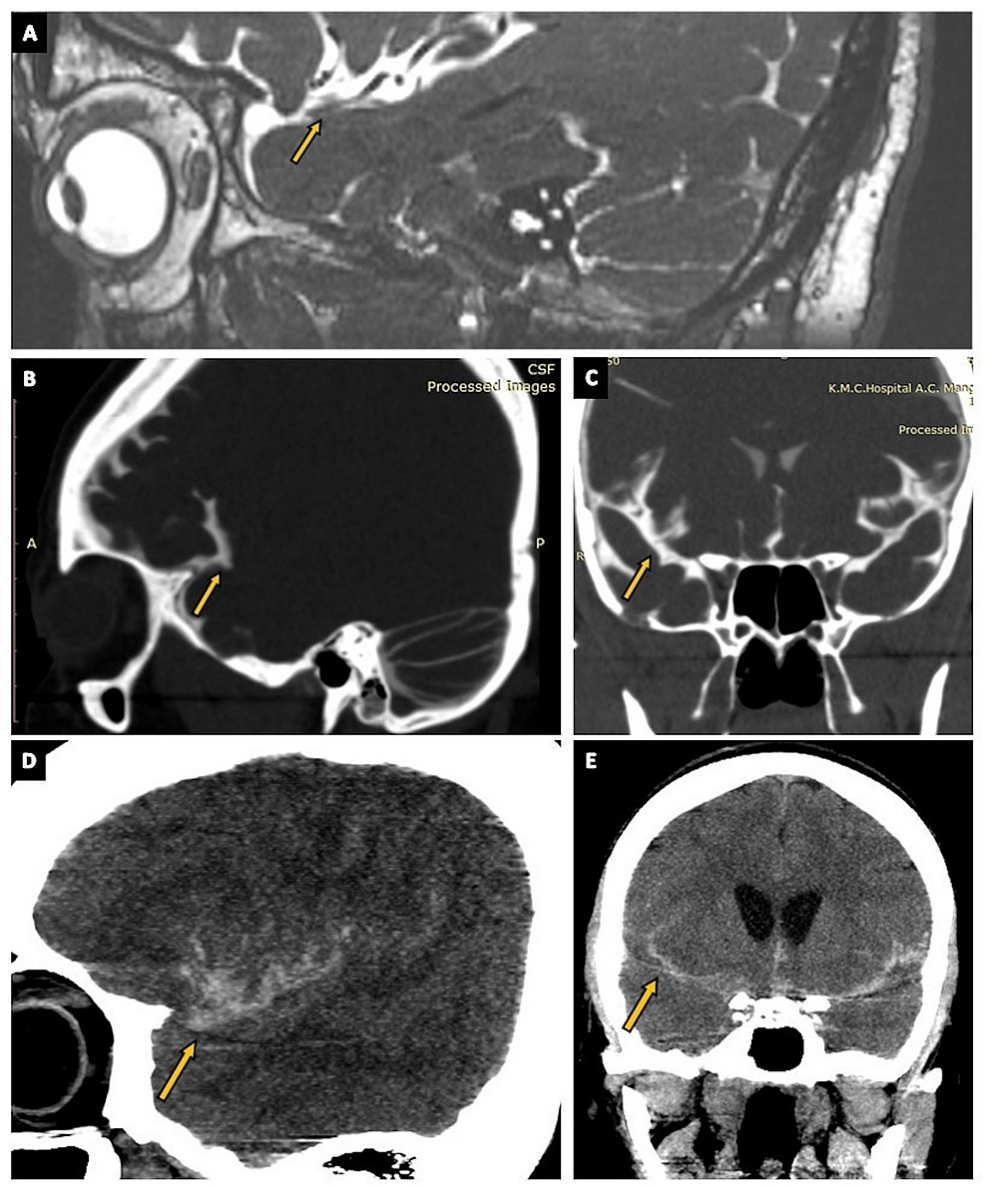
The Sylvian cistern. (A) The Sylvian cistern (arrow) as seen on a parasagittal section of the routine three-dimensional (3D) constructive interference in steady state (CISS) MRI sequence. (B) The Sylvian cistern (arrow) vizualised on a parasagittal section of CT cisternography in a case of suspected CSF rhinorrhea. (C) The Sylvian cistern (arrow) vizualised on a coronal section of CT cisternography in a case of suspected CSF rhinorrhea. (D) The Sylvian cistern (arrow) as seen on a parasagittal section of CT brain (positive contrast) in a case of subarachnoid hemorrhage (SAH). (E) The Sylvian cistern (arrow) as seen on a coronal section of CT brain (positive contrast) in a case of SAH.
